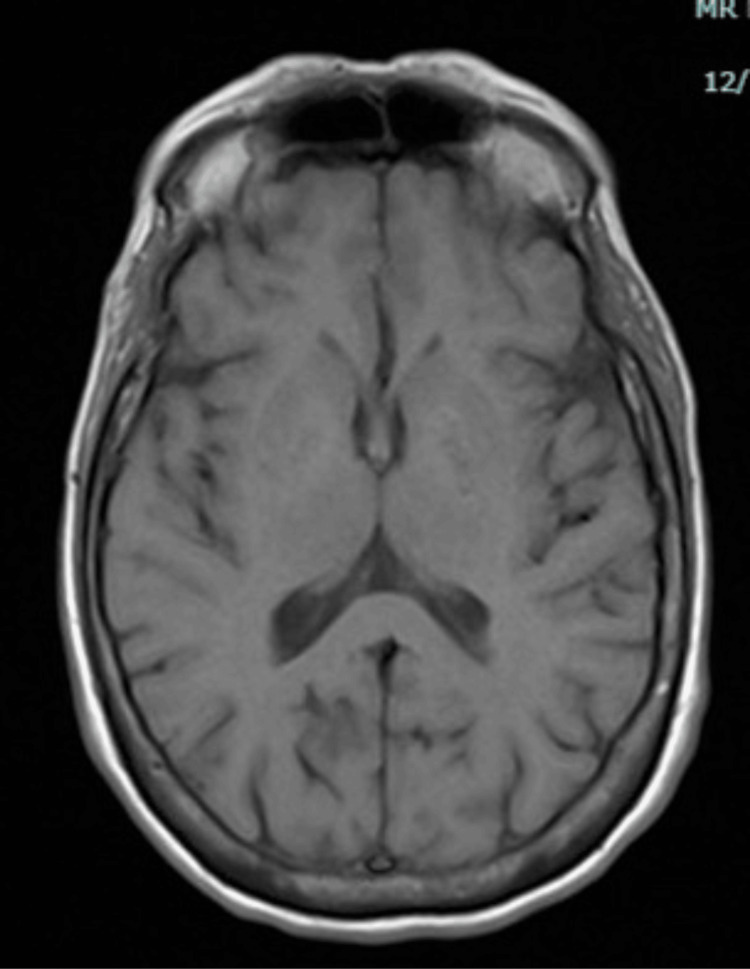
Axial T1-weighted MRI of the brain Mild chronic nonspecific white matter changes, most commonly reflecting chronic microvascular ischemic change. No acute intracranial abnormality identified.
